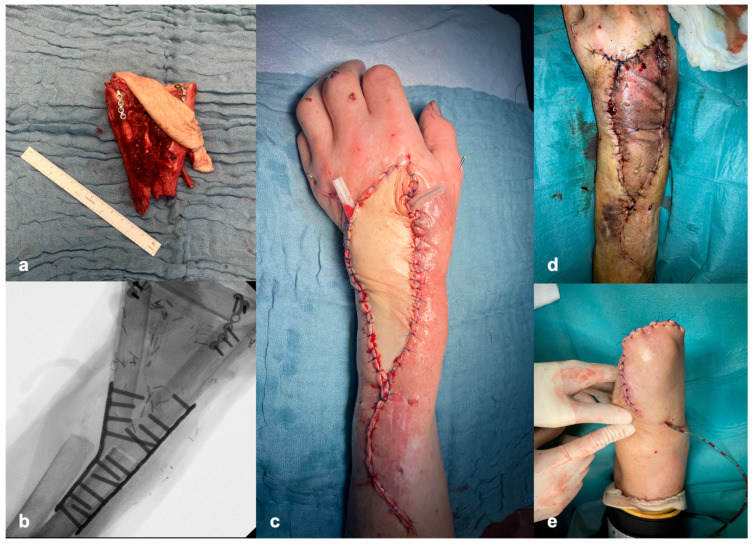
Reconstructive surgery. (a) Free osteocutaneous triple-barrel fibula flap. (b) X-ray of the wrist with radio-metacarpal reconstruction. (c) Clinical result after inset of flap. (d) Necrotic skin island eight days after reconstructive surgery. (e) Transradial forearm amputation.
